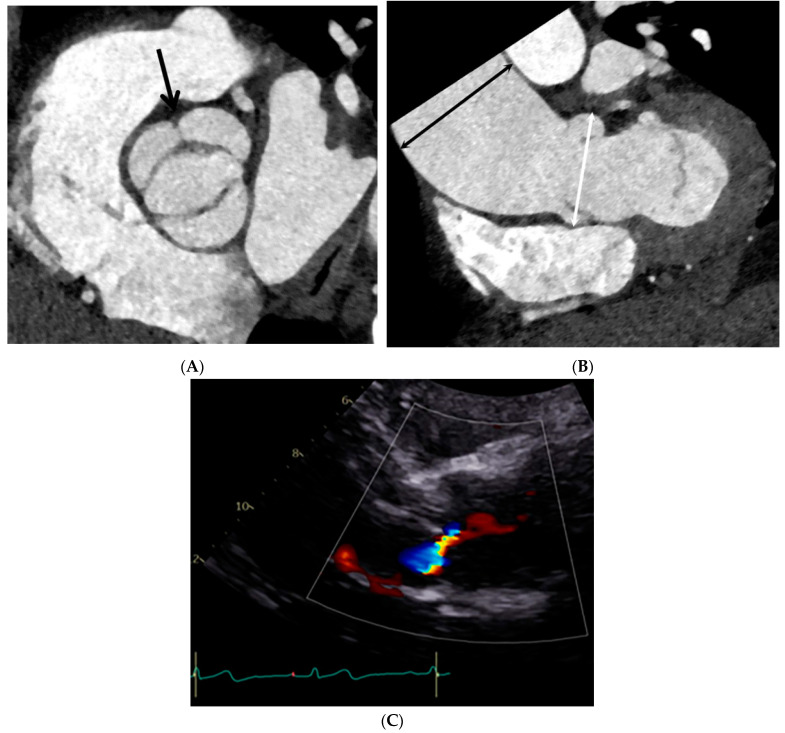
Bicuspid aortic valve (BAV) with a raphe with ascending aorta root dilatation in a 48-year-old male. (A) Double-oblique CT reconstruction parallel to the aortic valve during systole demonstrates a BAV with fusion of the right and left coronary cusps. (B) Double-oblique coronal CT reconstruction through the left ventricular outflow tract during systole shows the diameters of the sinuses of Valsalva (white arrow) at 40.8 mm (size index 2.02 cm/m^2^) mm and the mid-ascending aorta (black arrow) at 53.6 mm (size index 2.65 cm/m^2^), which were measured at mid-systole. (C) Color Doppler echocardiogram shows a jet regurgitating into the left ventricle. Aortic regurgitation was considered mild.
